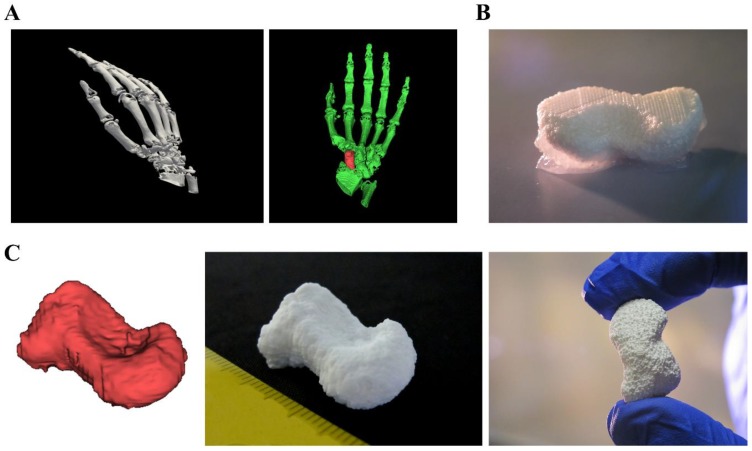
Fabrication of a scaphoid bone from clinical CT data. (A) 3D rendered human right hand and selection of the scaphoid by usage of 3D rendering software. (B) 3D multichannel plotting of the scaphoid bone consisting of CPC with mc10 as support ink. (C) Comparison of the stl-file and the real plotted structure with the characteristic shape of the human scaphoid.
